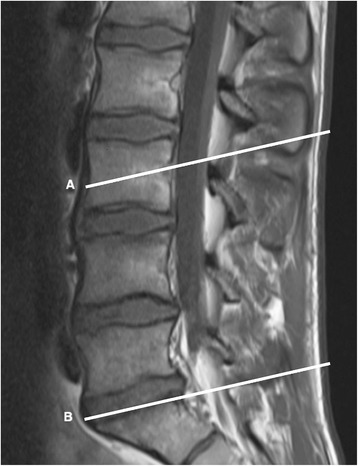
Sagittal view depicts the range (A-B) within axial MR images were analyzed
